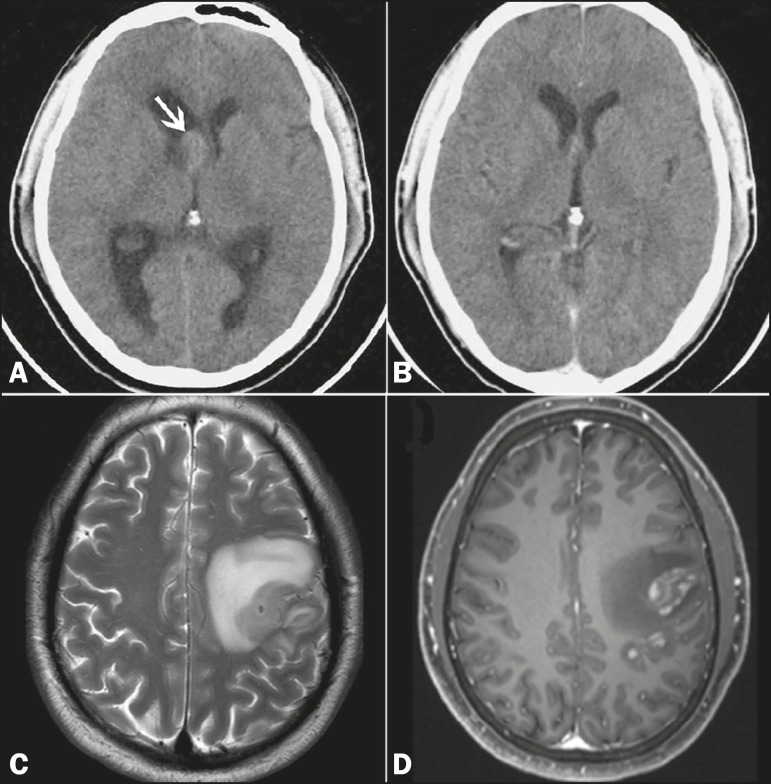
A: Non-contrast-enhanced CT scan of the brain, showing
